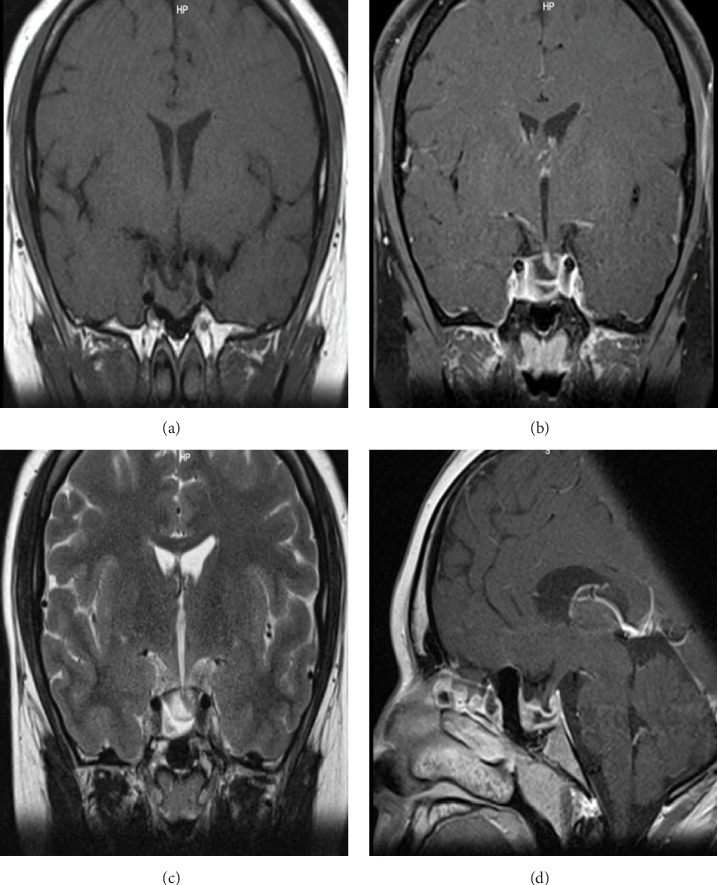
Pituitary MRI: (a) coronal T1-weighted, (b) coronal contrast-enhanced T1-weighted with gadolinium, (c) T2-weighted, and (d) sagittal T1-weighted. The images show a marked decrease in size of the known pituitary adenoma, measuring 10 mm (L) × 18 mm (T) × 18 mm (AP). The pituitary stalk is displaced laterally to the left. MRI, magnetic resonance imaging.
